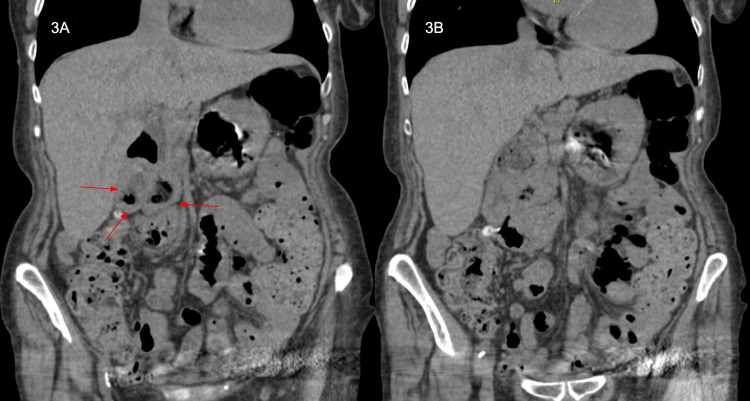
A-B: CT abdomen and pelvis without contrast in coronal view of intraluminal duodenal diverticulum (red arrows).
